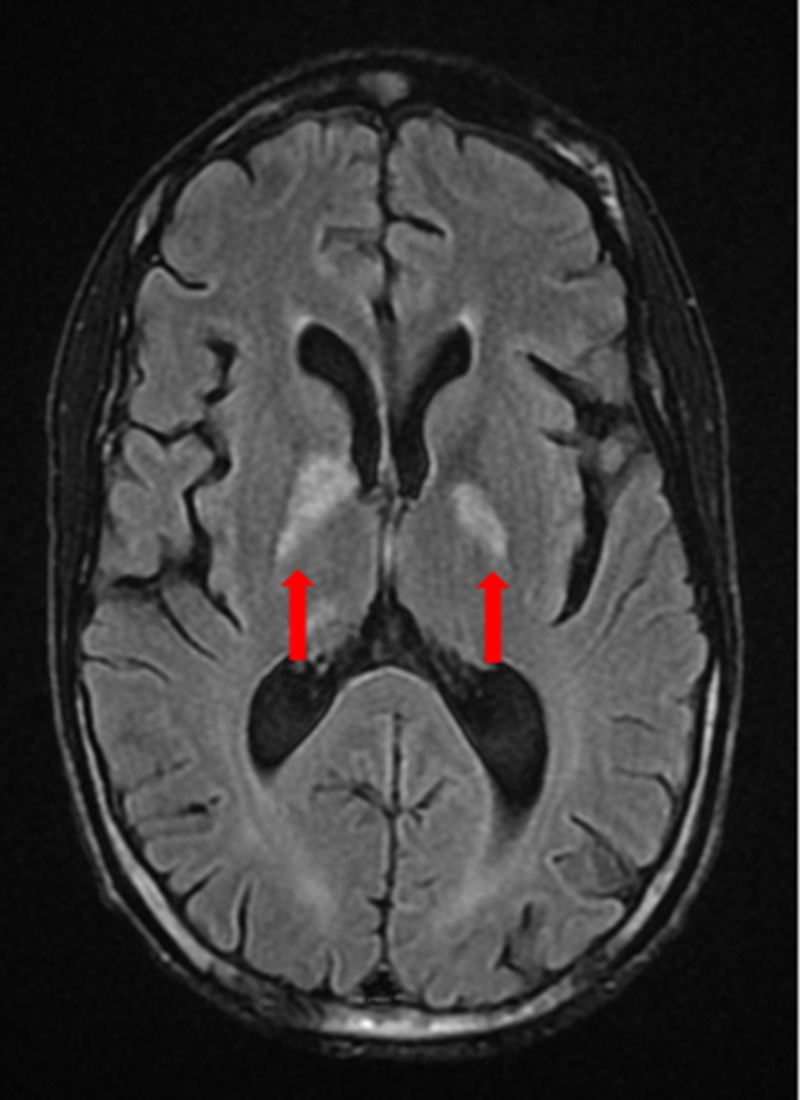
MRI of the brain with and without contrast The image shows acute/subacute infarcts (red arrows) MRI: magnetic resonance imaging
